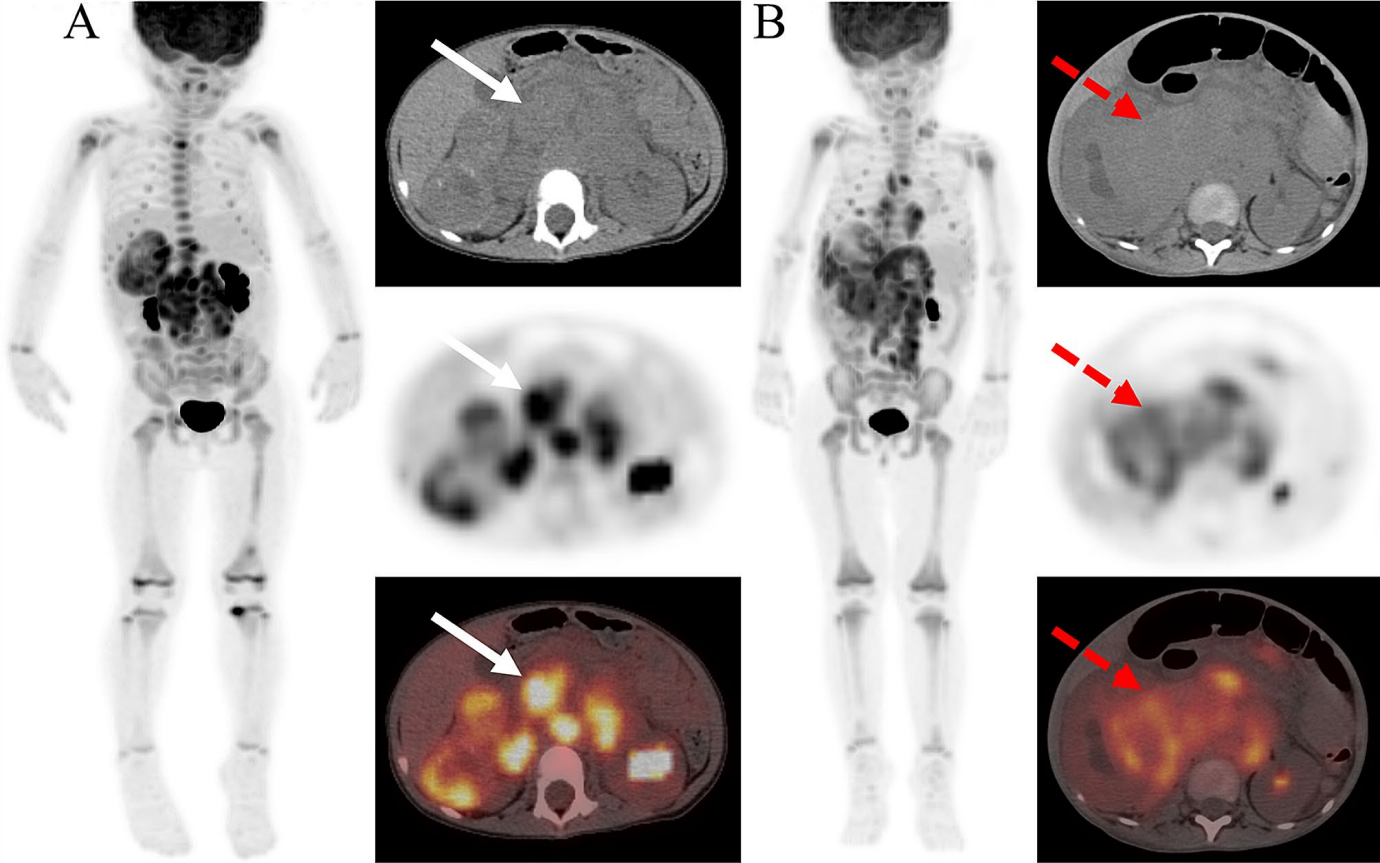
Two representative NB patients with high and low WMH values; A: a 32-month-old girl with a relatively uniform FDG uptake in whole-body tumor (White arrow, IMH:0.443, WMH:0.470), was disease free survival 51.3 months after diagnosis; B: a 29-month-old girl with a heterogeneous FDG uptake in whole-body tumor (Red arrow, IMH:0.463, WMH:0.392), relapsed at 7.1 months, and died at 8.9 months after diagnosis
